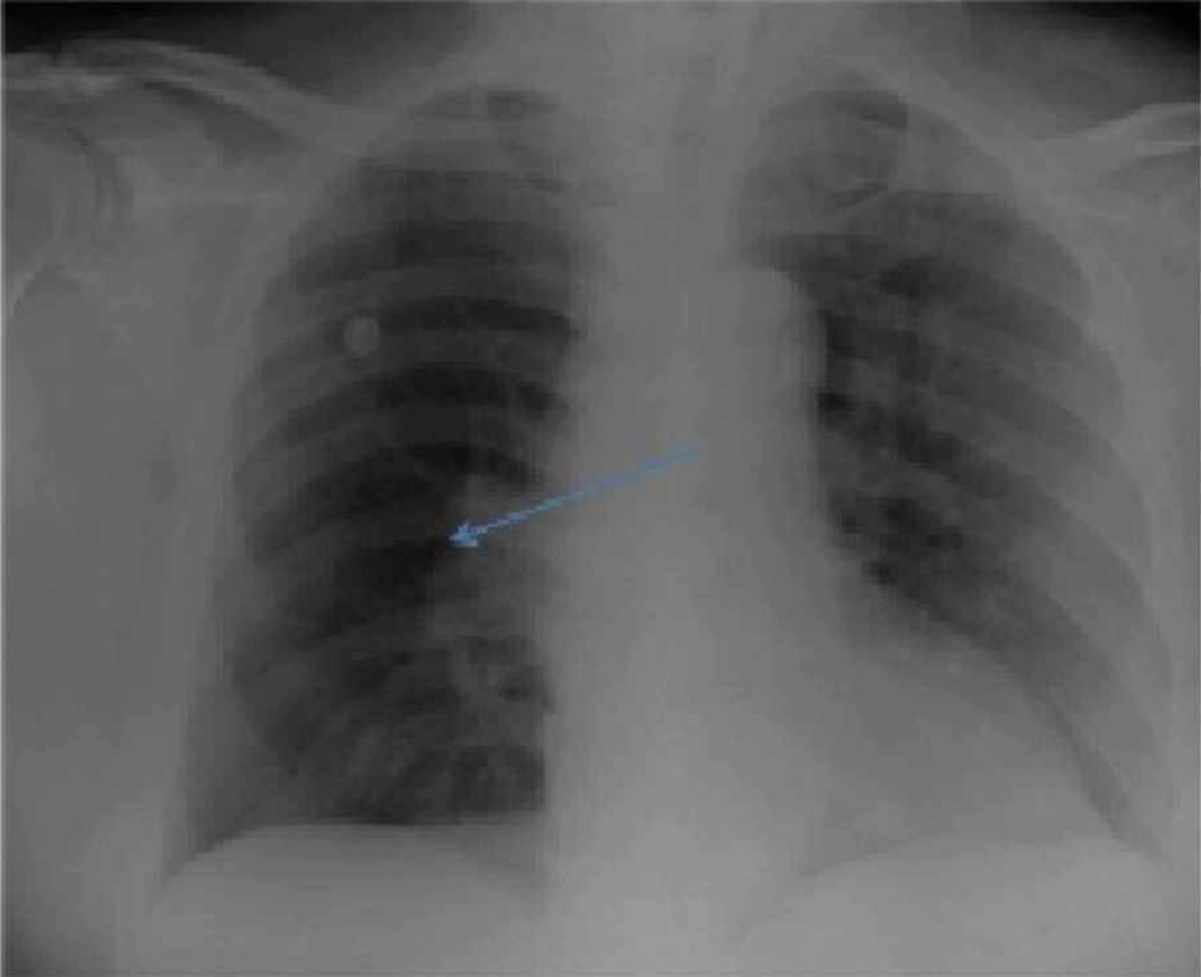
Initial portable anteroposterior chest X-ray (CXR) showing right-sided hilar vascular prominence for dilated pulmonary artery (Fleischner sign) and lung field oligemia, especially evident on the right side (Westermark sign)along with an abrupt absence of distal vessels.
